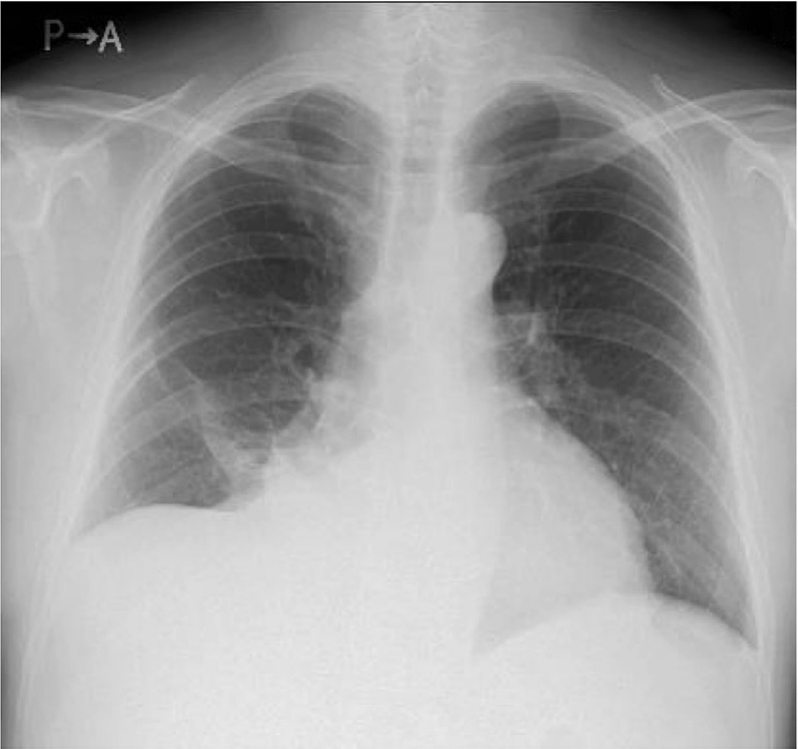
Chest X-Ray There is right slight pleural effusion and cardiomegaly.
